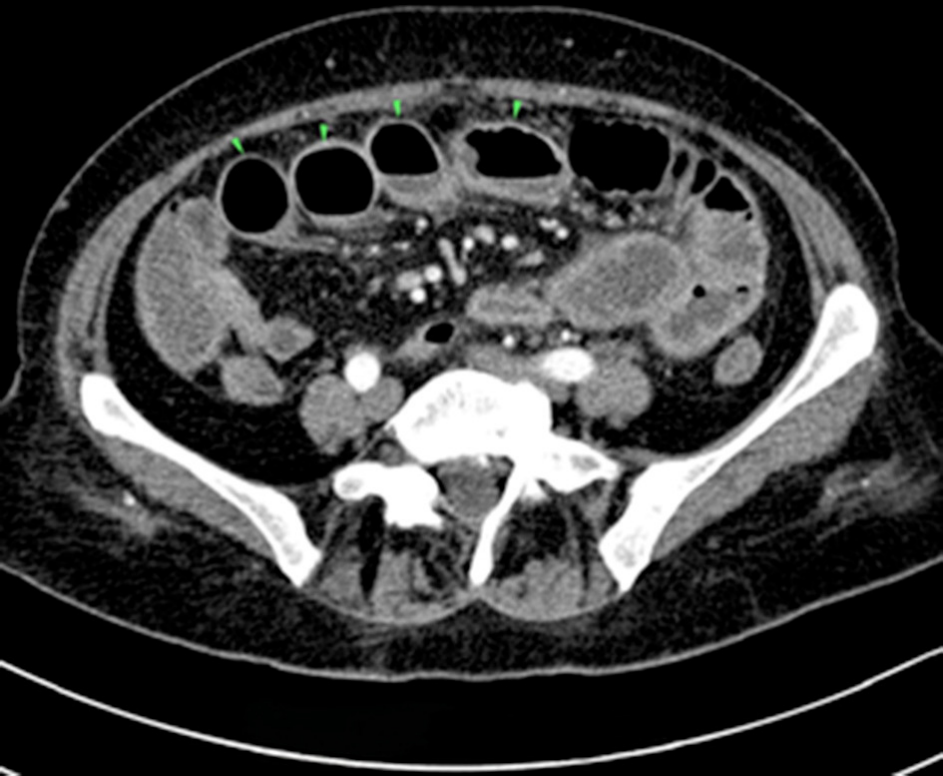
Computed tomography of the abdomen and pelvis. Dilated ileal loops (arrowheads).
